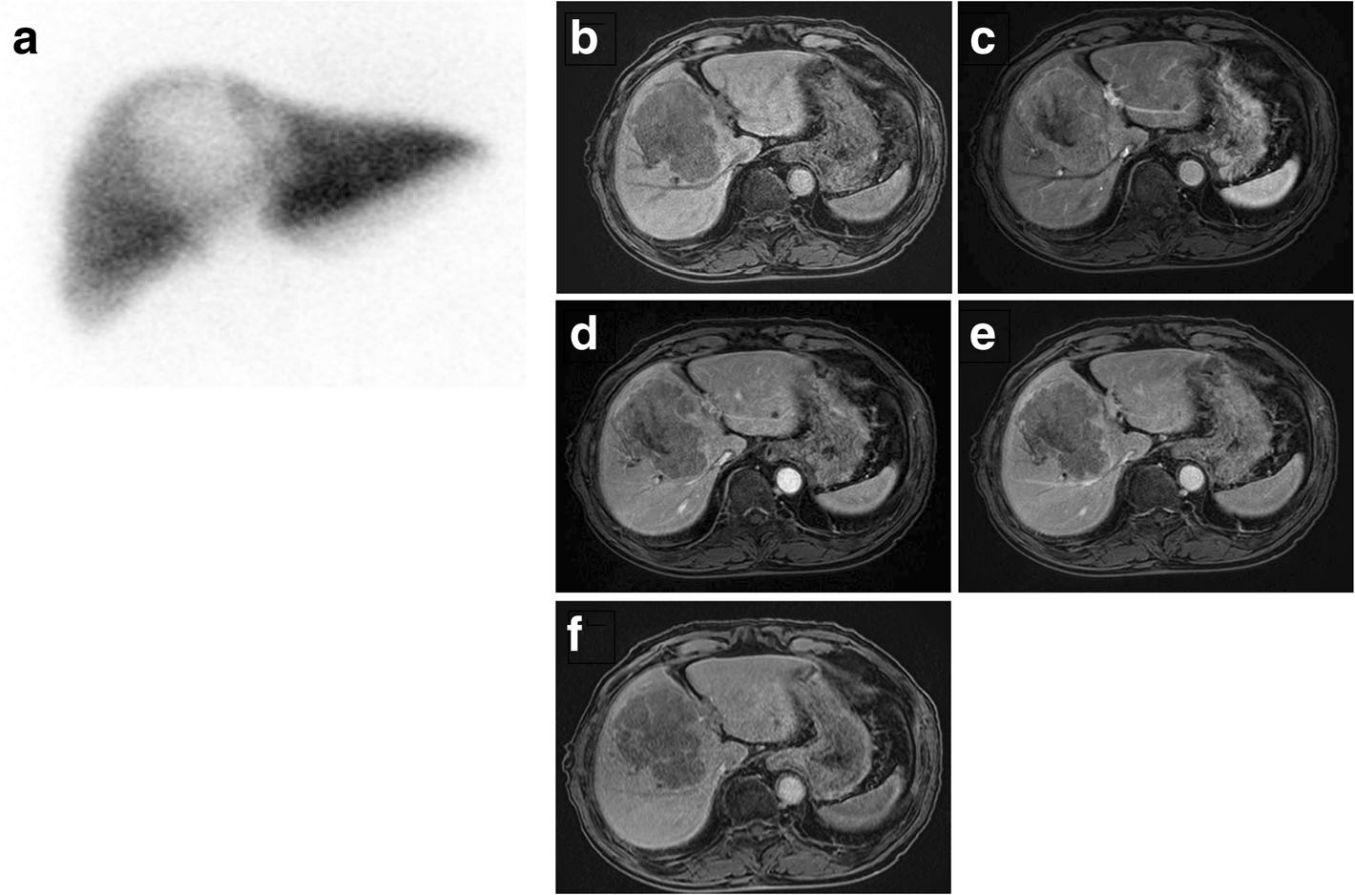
^99m^Tc-DTPA-galactosyl human serum albumin (GSA) liver scintigraphy showed normal accumulation of GSA in non-neoplastic lesion (a). Each gadolinium-ethoxybenzyl-diethylene triamine pentaacetic acid (Gd-EOB-DTPA)-enhanced magnetic resonance imaging (MRI) showed pre-contrast T1-weighted image (b), arterial dominant phase (c), portaldominant phase (d), transitional phase (e), and hepatobiliary phase (f). Hepatobiliary phase showed slight enhance with poor contrast enhancement to spleen or vessels in non-neoplastic site
